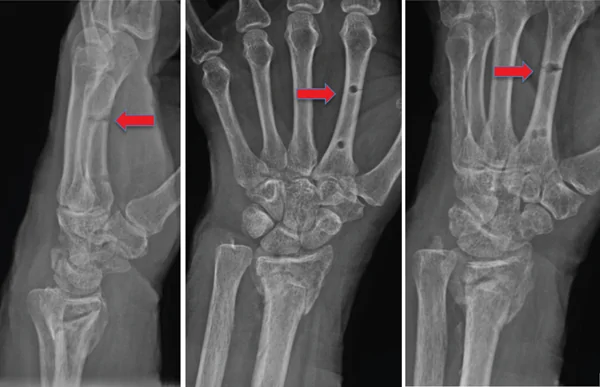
Radiographs taken immediately following external fixator, pin, and wire removal, demonstrating nondisplaced 2nd metacarpal shaft fracture across the pin site.
